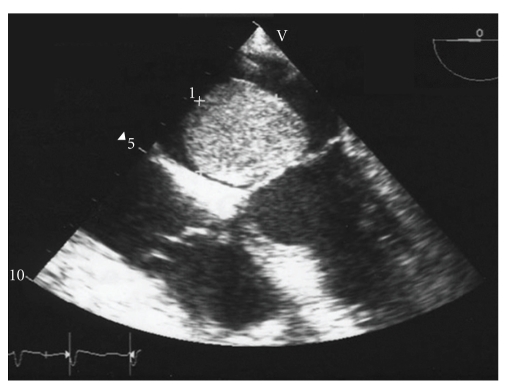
Transesophageal echocardiography revealing large heterogeneous density tumor in the left atrium.
